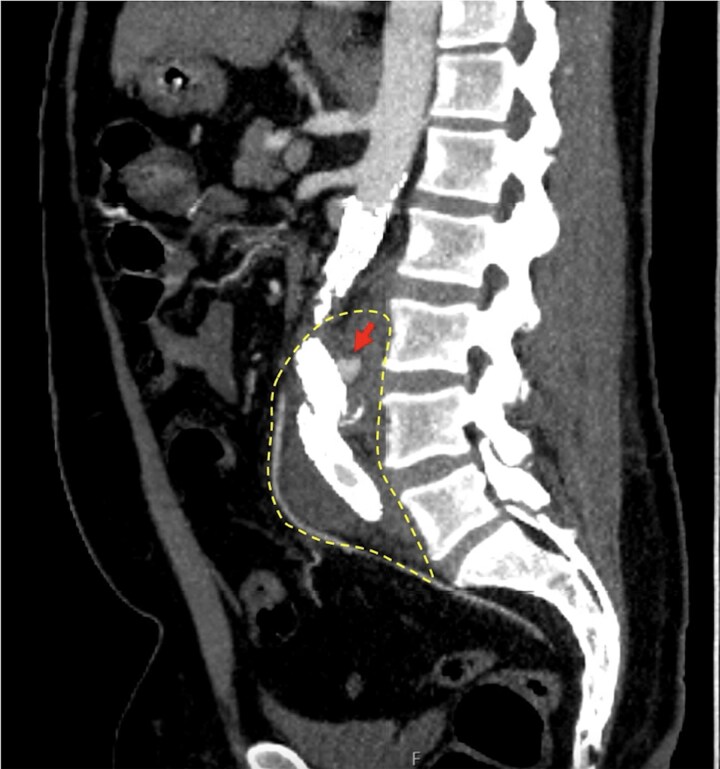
Case 1: sagittal cut of computed tomography angiogram demonstrates contrast extravasation (arrow) at the junction of the left iliac limb of the endovascular aneurysm repair, suggestive of a type III endoleak. Perigraft soft tissue stranding (dashed circle) is suggestive of phlegmon.
